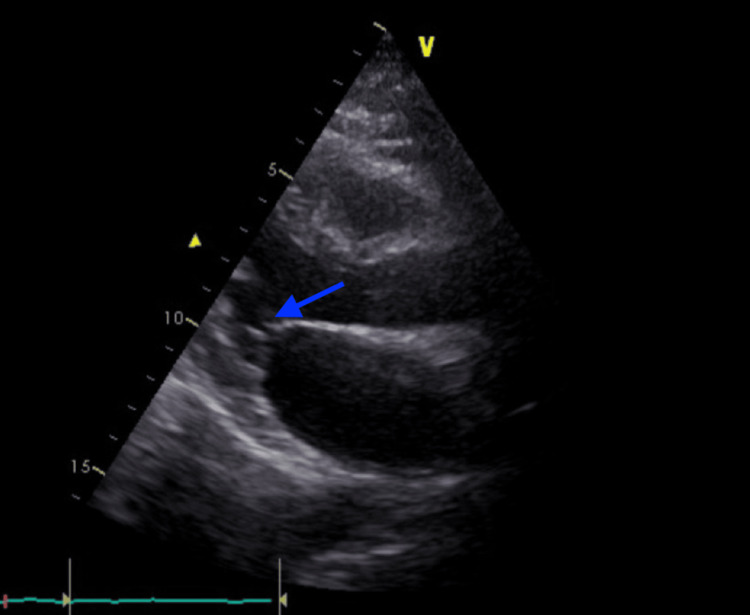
TTE performed four weeks after treatment initiation showing complete resolution of the endocarditis The disappearance of vegetations on the mitral valve is indicated (blue arrow). TTE, transthoracic echocardiogram
